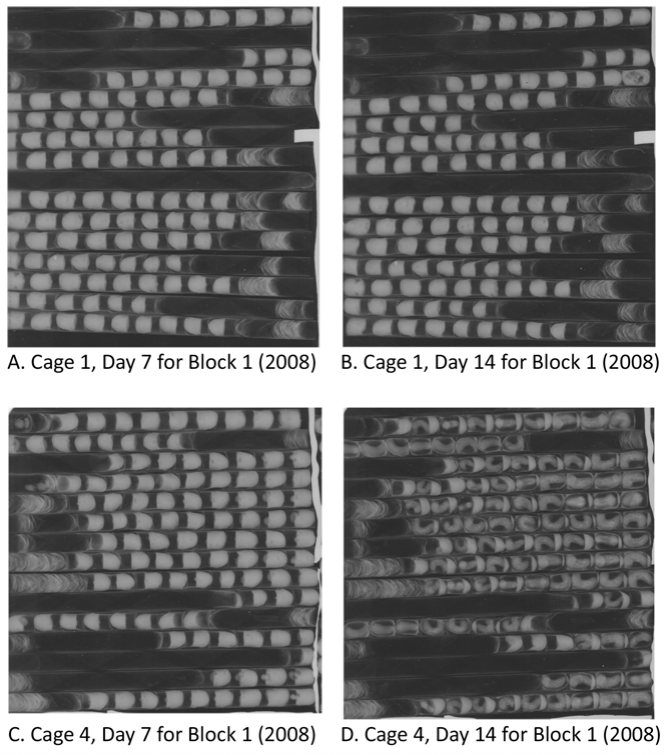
Example of x-rayed paper straws with *Megachile rotundata* cells. Note the absence of larval development after 14 days when adults are fed novaluron for 24 hours (B) compared to when adults are not exposed (D). High quality figures are available online.
